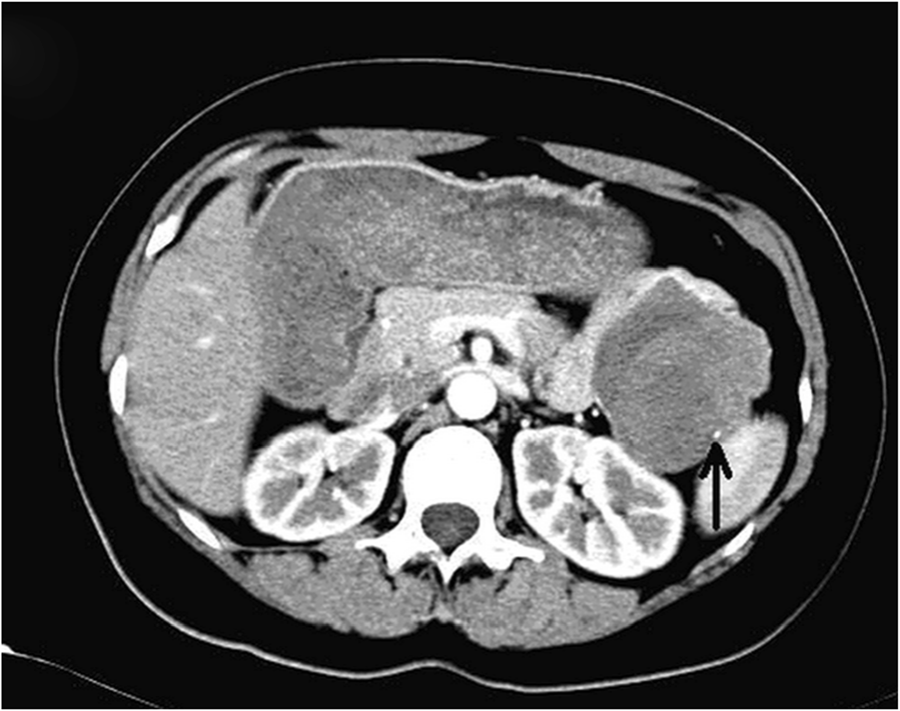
Contrast-enhanced CT shows a solid and cystic mass with a calcification (arrowed) located in the tail of the pancreas.
